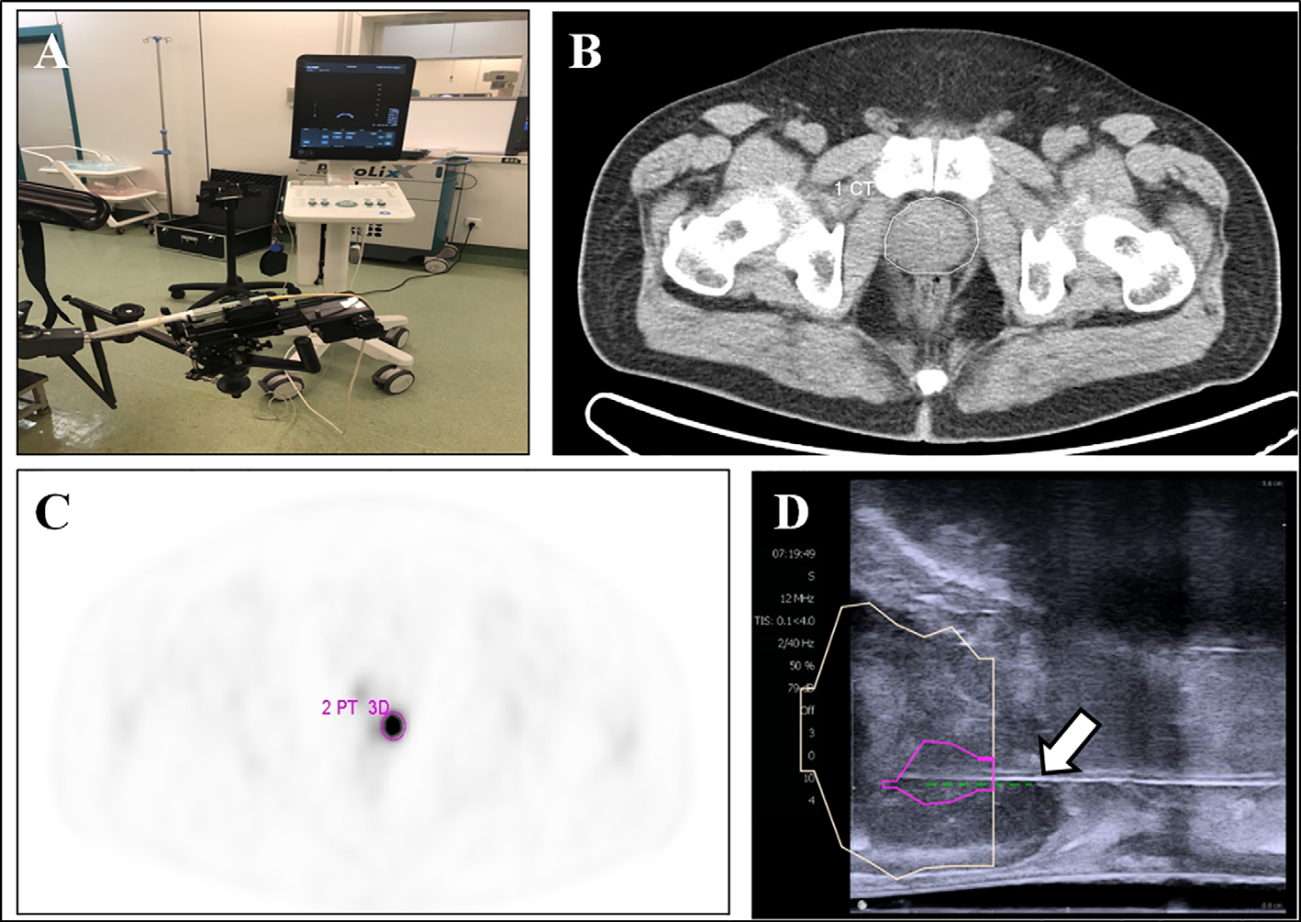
^18^F-DCFPyL PET/CT-US or PET/MRI-US fusion targeted prostate biopsy for the intraprostatic PET-positive lesions were performed with the BK Predictive Fusion prostate biopsy system (A). The boundaries of the prostate were delineated on the CT image (B, white circle). The PET-positive lesion was marked as the target for biopsy (C, pink circle). The previous delineated prostate and PET-positive lesion from PET/CT was registered to the prostate volume acquired from the three-dimensional transrectal ultrasonography; the puncture needle (D, arrow) then reached the target biopsy area.
